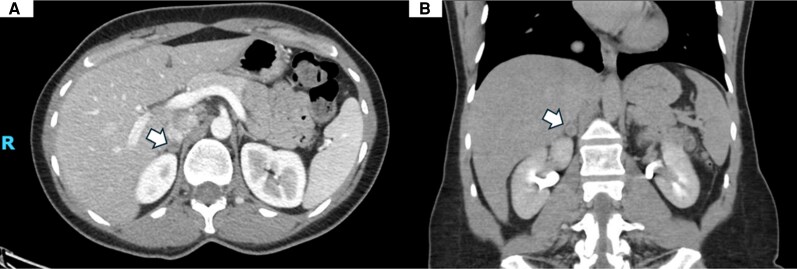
Repeat computed tomography of the abdomen with intravenous contrast at 1-year follow-up. The arrows show a 1.0 × 1.0 cm in the axial view (5A) and coronal view (5B) demonstrating an interval decrease of the adrenal lesion.
